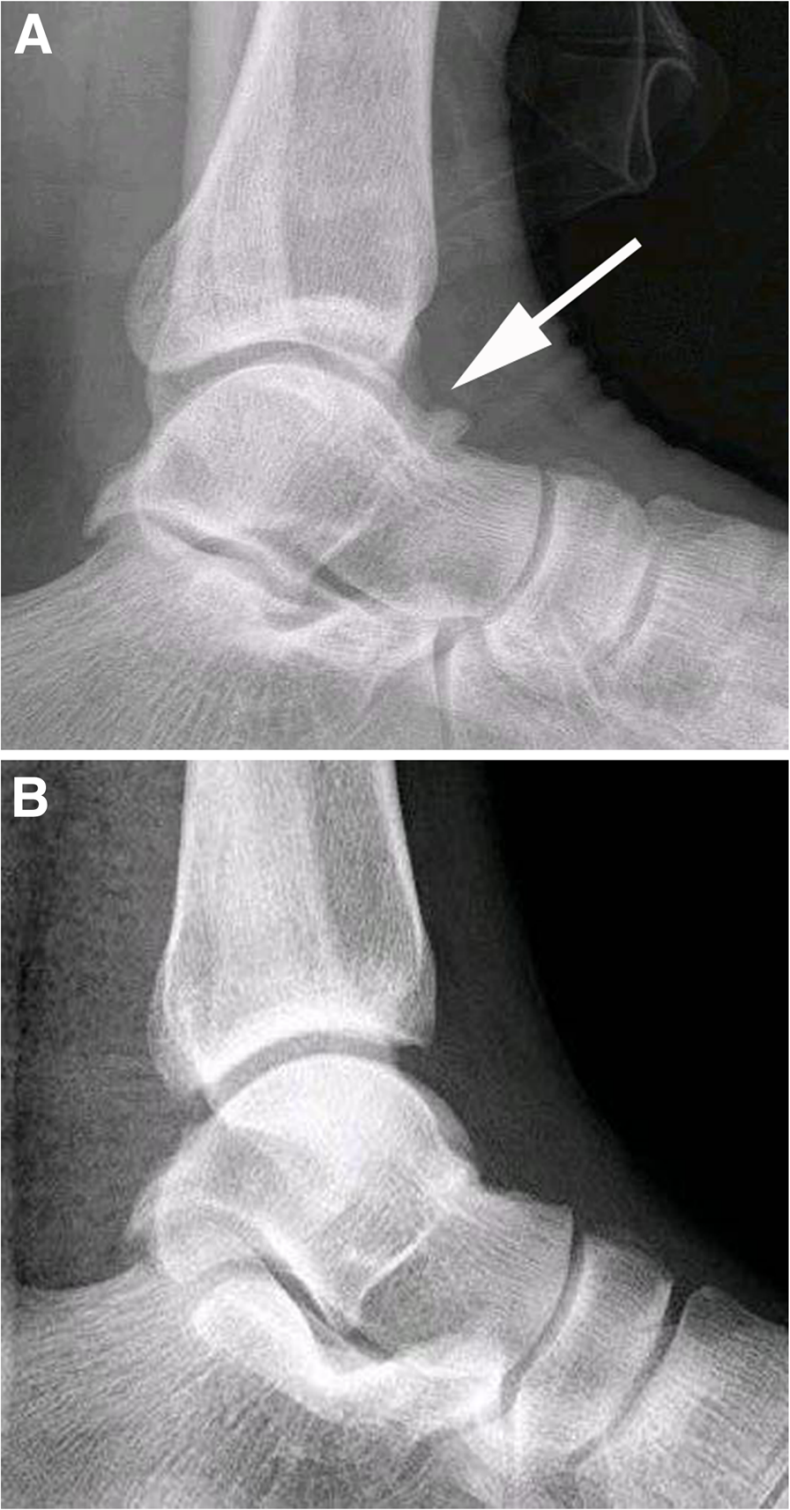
X-ray images of an ankle with bony anterior ankle impingement preoperatively (a) and postoperatively (b). White arrow indicates osteophytes
